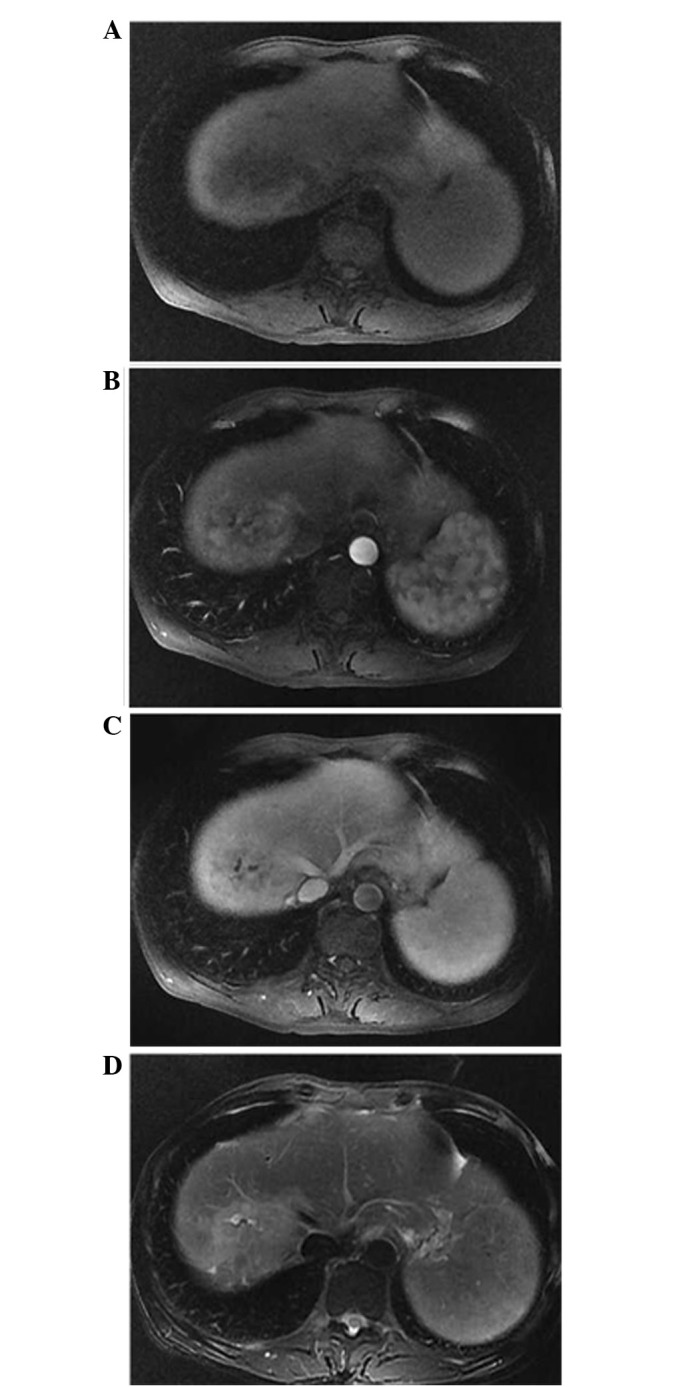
Magnetic resonance imaging (MRI) demonstrating that the inflammatory pseudotumor was (A) hypointense on the unenhanced T1-weighted image, (B) partially hyperintense on the enhanced T1-weighted image, (C) hypointense on the hepatobiliary phase image (T1-weighted) and (D) partially hyperintense on the unenhanced T2-weighted image.
